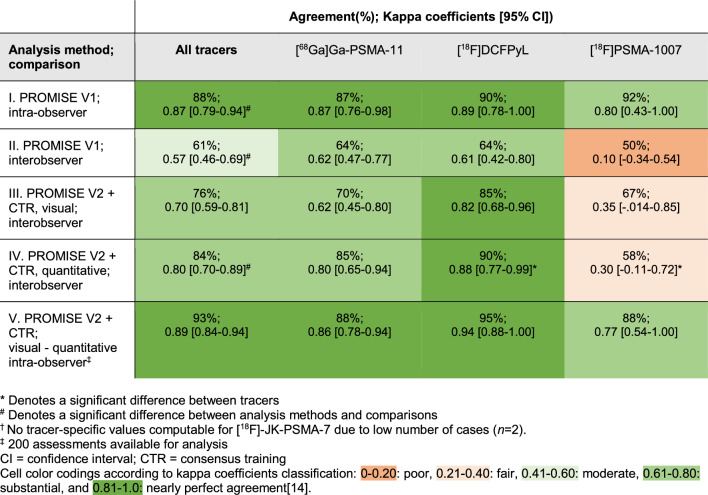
Overall and tracer-specific^†^ agreement of PSMA-expression scores in 100 patients undergoing pre-operative staging PSMA PET/CT in primary prostate cancer.
